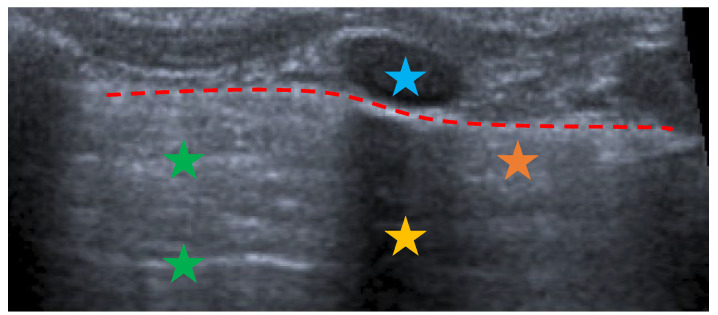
Ultrasound image corresponding to healthy lung tissue. The different structures and ultrasound artefacts can be seen: ribs (blue) causing a posterior acoustic shadow (yellow), pleural line (red), A-lines (green) and B-lines in normal amount (orange).
